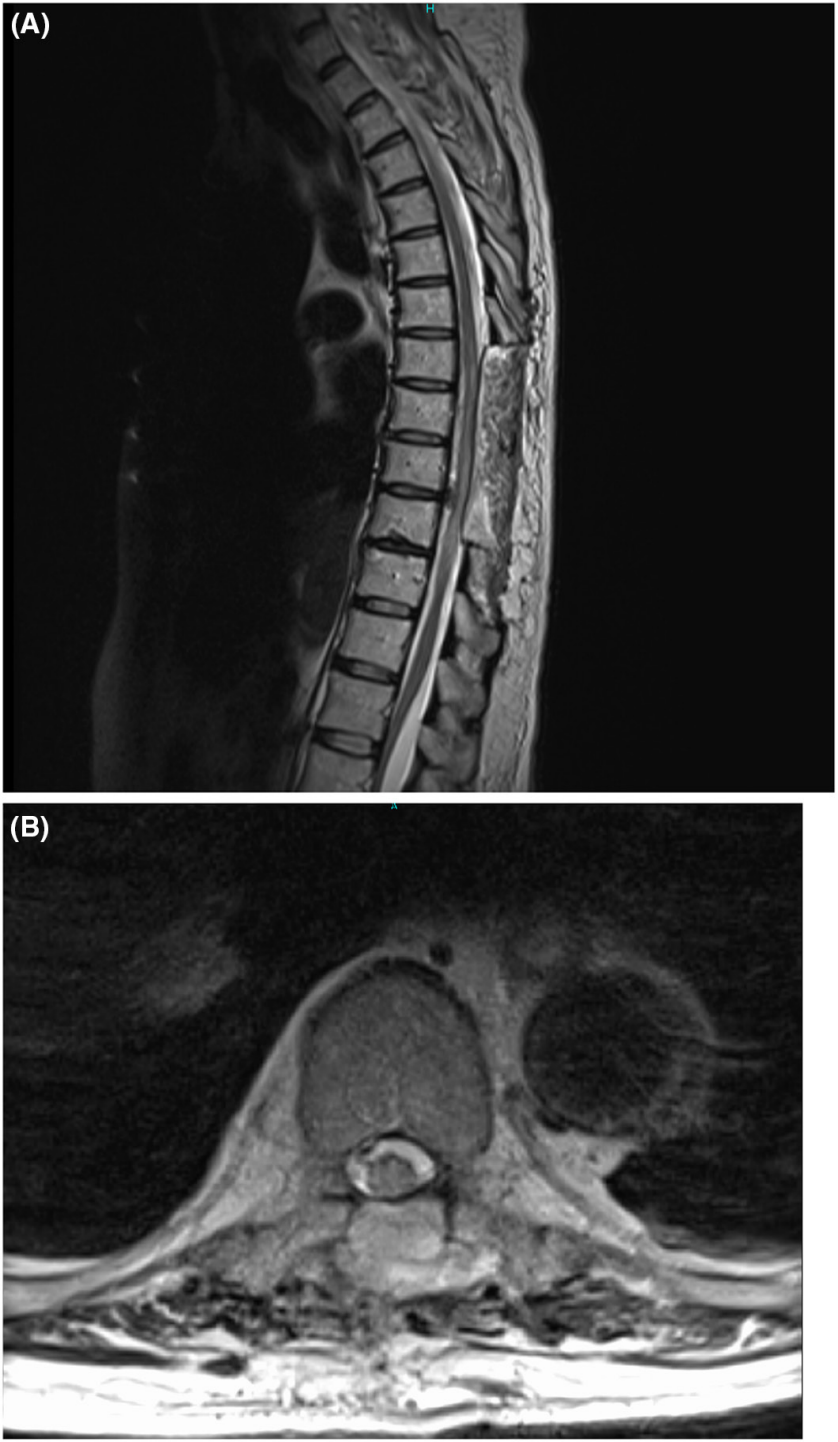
MRI 2 weeks after surgery. (A) Sagittal T2‐weighted MRI revealed that the compression of the spinal cord due to hematoma was relieved from the T7 level to the T10 level. (B) Axial T2‐weighted MRI also showed that spinal cord compression at the T10 level was relieved.
